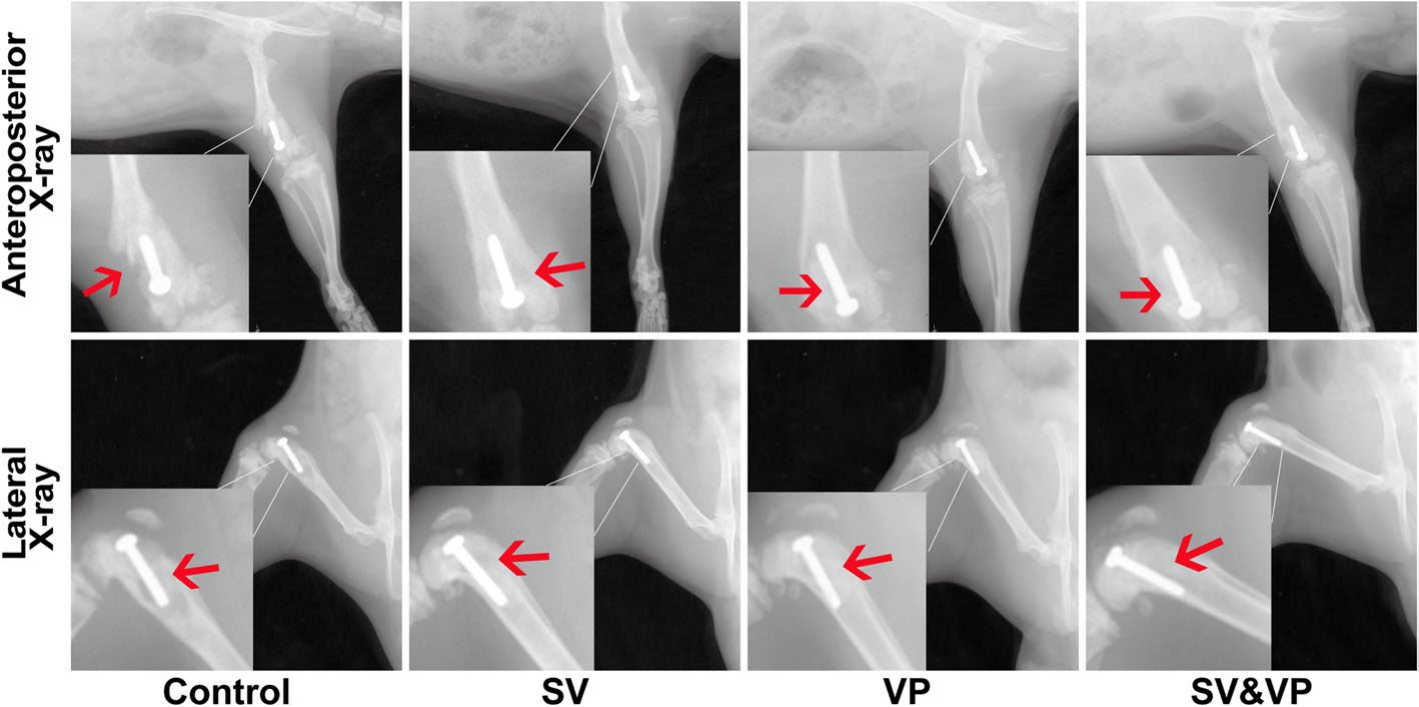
X-ray evaluation of the knee joint and prosthesis on days 28. Control (no antibiotics); SV: Systemic vancomycin (intraperitoneal injection, 88 mg/kg, every 12 h); VP: Intra-wound vancomycin powder (before closure of the capsule, 88 mg/kg, single dosage); SV&VP: Systemic vancomycin & intra-wound VP (intraperitoneal injection, 88 mg/kg, every 12 h; combined with intra-wound VP before closure of the capsule, 88 mg/kg, single dosage). The red arrow indicates the position of prosthesis and destruction of the bone
